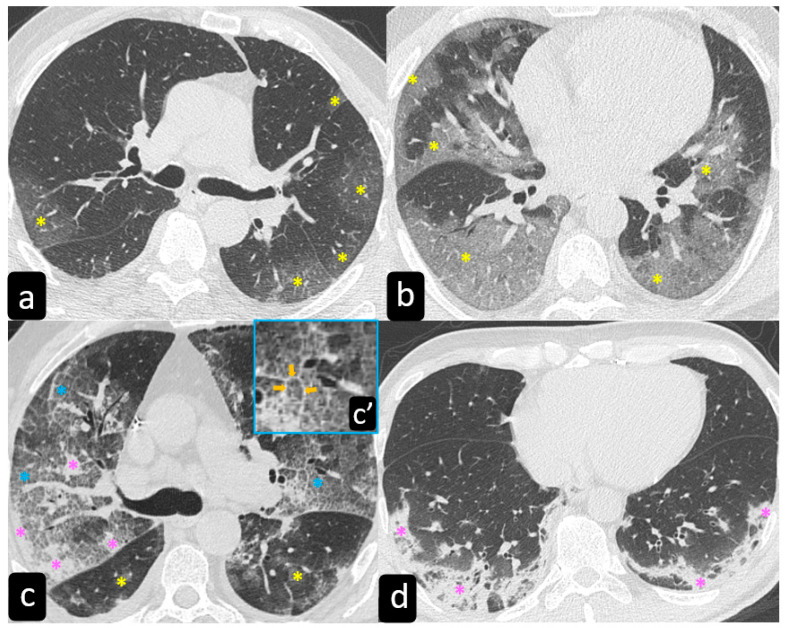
HRCT findings of COVID-19 pneumonia. Axial HRCT scans show bilateral ground glass opacities (yellow * in (a–c)) with or without posterior and peripheral lung consolidation (pink * in (c,d)) and “crazy-paving” pattern (blue * in (c)). “Crazy-paving” refers to the presence of ground-glass opacity with superimposed interlobular septal thickening (orange arrows in (c’)).
